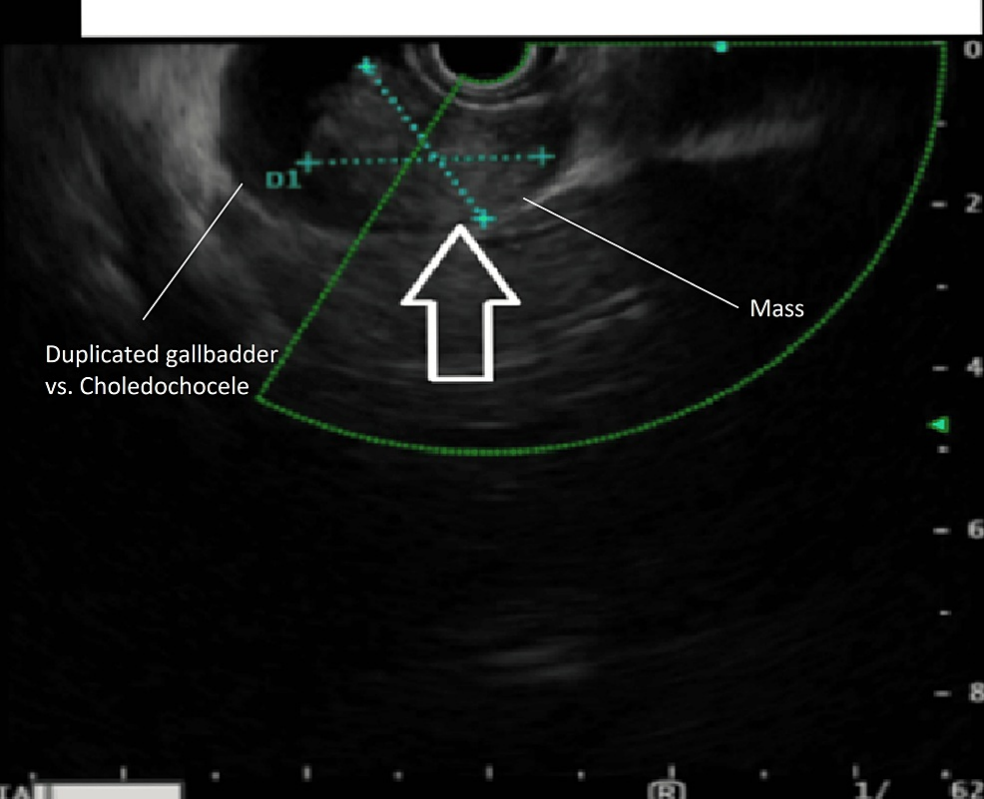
EUS with an arrow pointing to an ovoid mass that appears to be arising from a choledochocele versus duplicated gallbladder. EUS, endoscopic ultrasound
